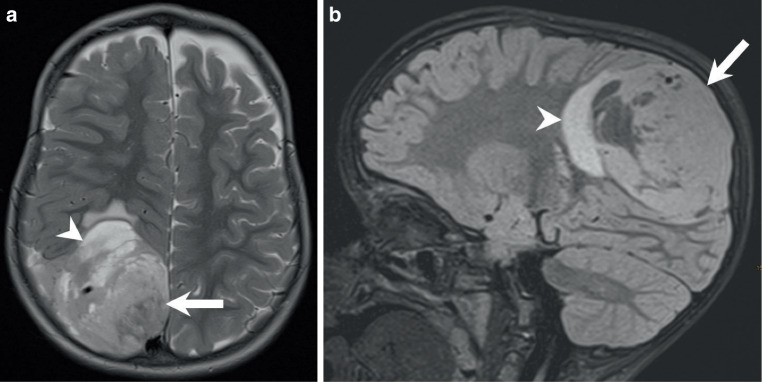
Axial T2-weighted MR images (a) showed a large right parietal lesion with a solid component (arrow) adjacent to cystic portions (arrowhead). On sagittal fluid-attenuated inversion recovery (FLAIR) images (b) the lesion appears isointense compared to the cerebral cortex (arrow). Surrounding perifocal edema is evident (arrowhead)
